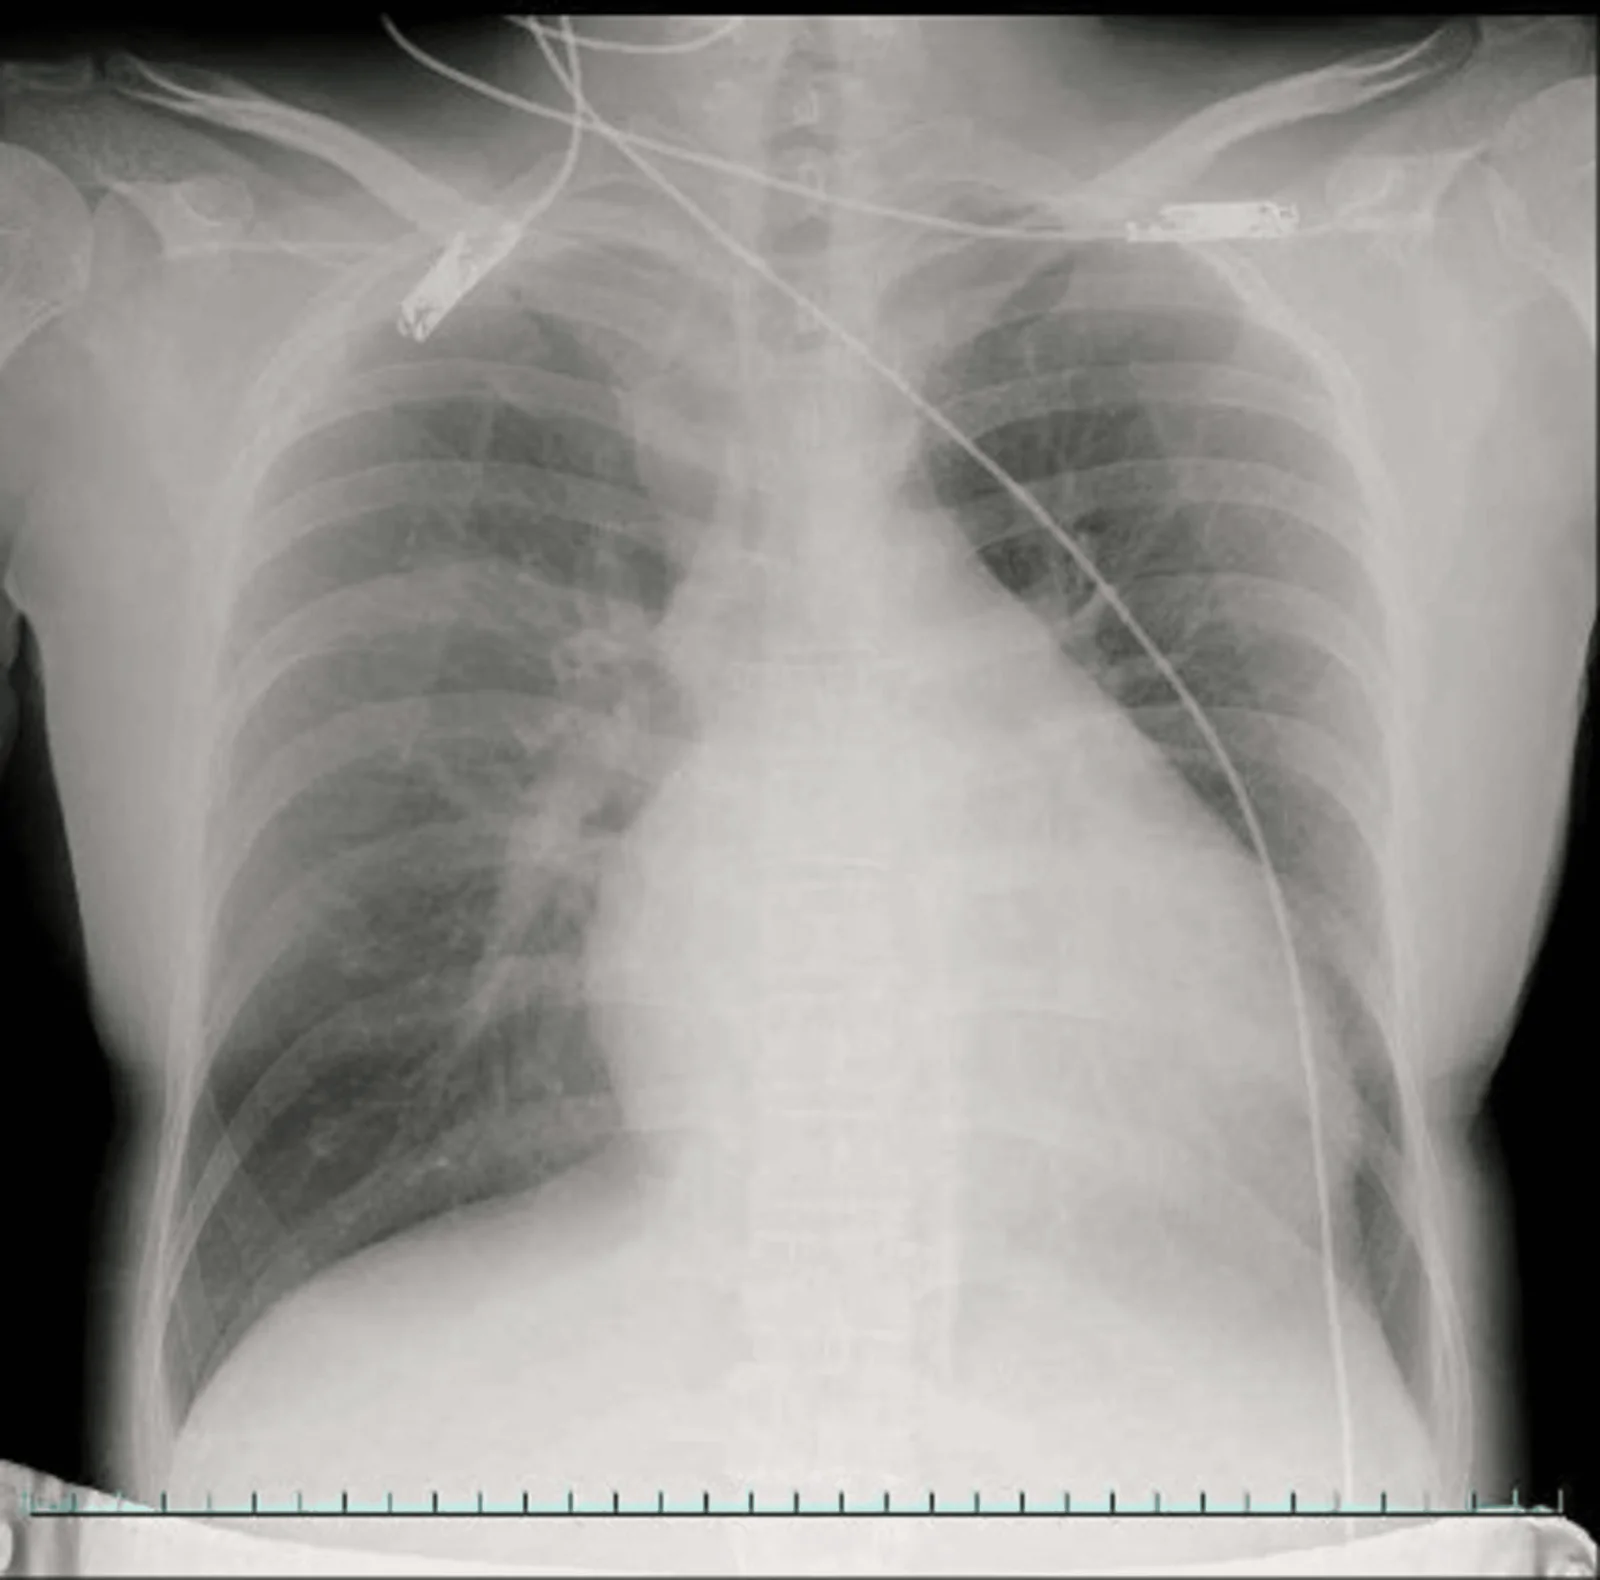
Chest radiograph on admission Chest radiography demonstrated marked cardiomegaly with a cardiothoracic ratio of 61.1% and pulmonary vascular congestion.
